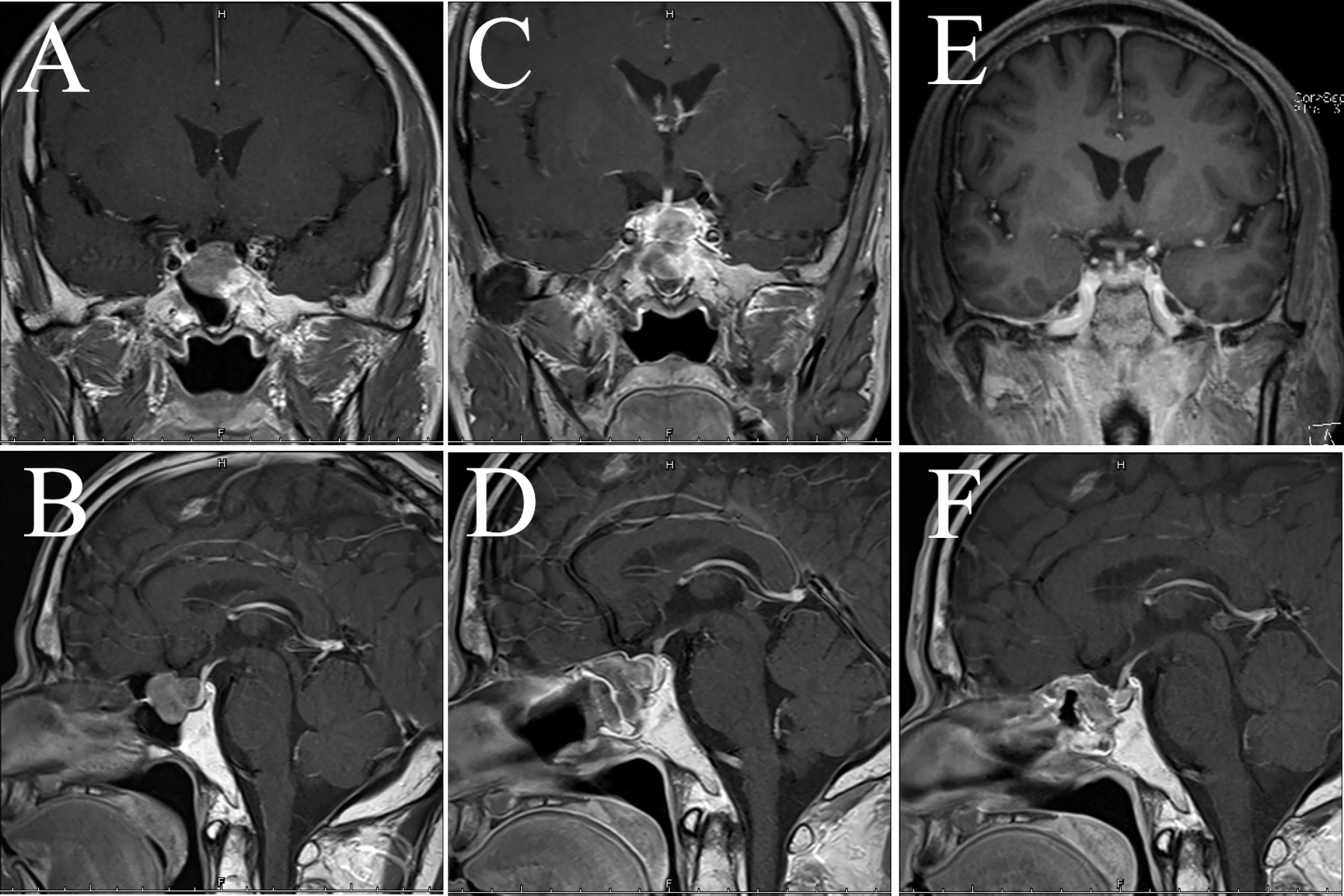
MRI examination of case 3. The images show coronal and sagittal MRI of the patient’s head preoperatively (A, B), one week postoperatively (C, D), and three months postoperatively (E, F), respectively.
